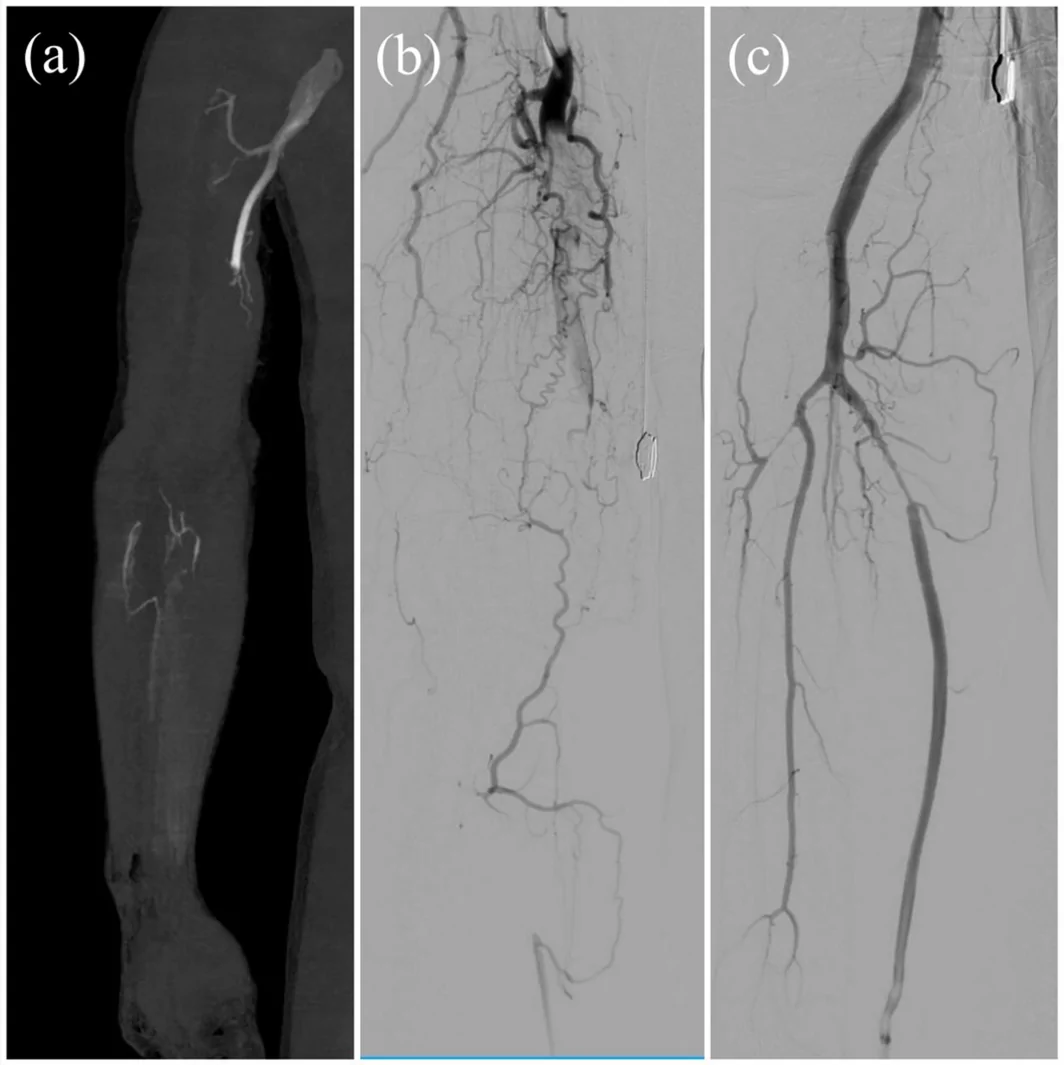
Preoperative CTA and intraoperative angiograms of the first endovascular procedure. (a) Preoperative CTA: Right axillary artery aneurysm with mural thrombus and total occlusion of distal brachial, radial and ulnar arteries; (b) intraoperative angiography before thrombus aspiration: Diffuse thrombotic occlusion of the right upper limb distal arterial tree; (c) post‐aspiration angiography: Restored patency of brachial, radial and ulnar arteries.
